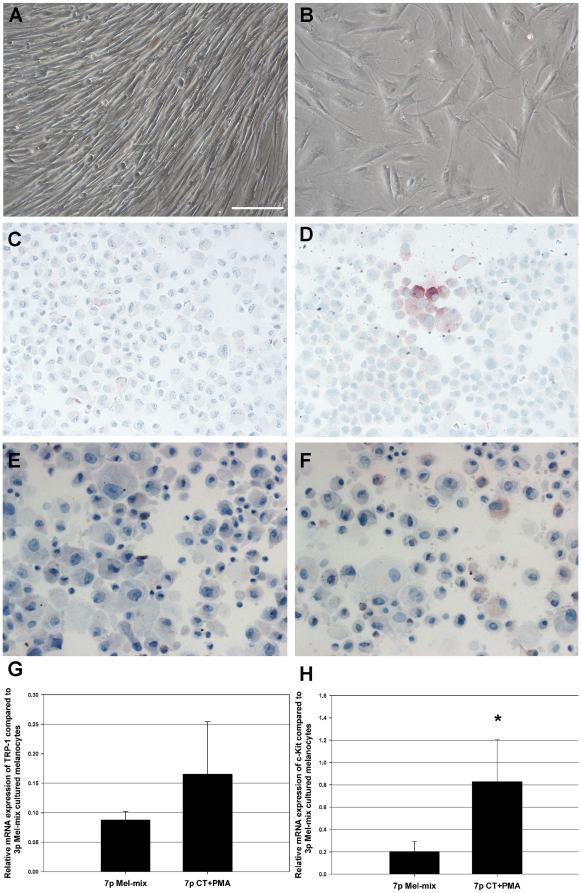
CT and PMA induce dendrite formation, TRP-1 protein and c-Kit mRNA and protein expressions in dedifferentiated melanocytes. Cultured, 7^th^ passage melanocytes showed a uniform, bipolar morphology in Mel-mix medium (A), while treatment of melanocytes with CT and PMA induced dendrite formation (B). CT+PMA induced both TRP-1 (D) and c-Kit (F) protein expressions in melanocytes compared to untreated cultures (C and E) as demonstrated by immunocytochemistry. Bar: 100 µm. At the mRNA level, CT and PMA caused a 2-fold increase in TRP-1 (G) and 4-fold increase in c-Kit (H) expressions compared to untreated controls. Values are shown as relative expressions compared to 3^rd^ passage Mel-mix cultured cells (mean±SD from three independent experiments; *significant at p≤0.05, Univariate ANOVA).
